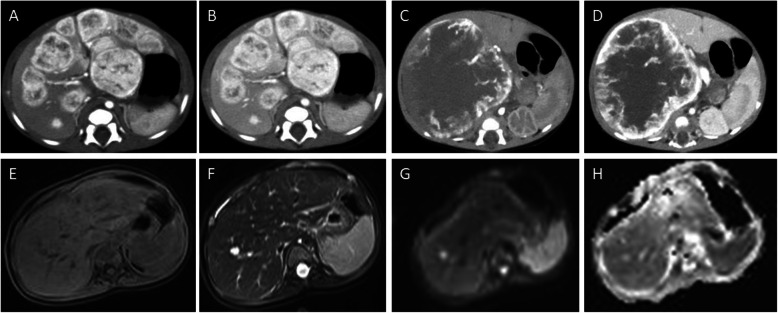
Typical cases of IHE. A 2-month-old female patient with IHE. The enhanced CT images display multiple hepatic lesions with markedly heterogeneous enhancement (a), gradually filling from the periphery to the center (b). A 2-month-old male patient with IHE. The enhanced CT images show a very large hepatic lesion with markedly marginal enhancement with nourishing blood vessels (c), gradually filling from the periphery to the center (d). A 7-month-old female patient with IHE. The MR images show hypointense lesions in the right hepatic lobe on T1WI (e), hyperintensity on T2WI (f), and hyperintensities on DWI (g) and the ADC map (h)
